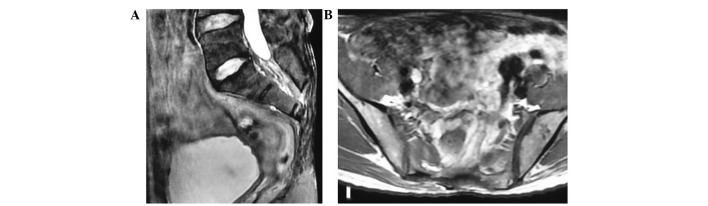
The tumor was marginally resected while preserving the bilateral S2 nerve roots. (A) MRI at the four-year follow-up showing no tumor recurrence (T1-weighted MRI, sagittal view). (B) Transverse view of MRI showing no recurrence in the surrounding muscle tissue (T2-weighted MRI, axial view).
